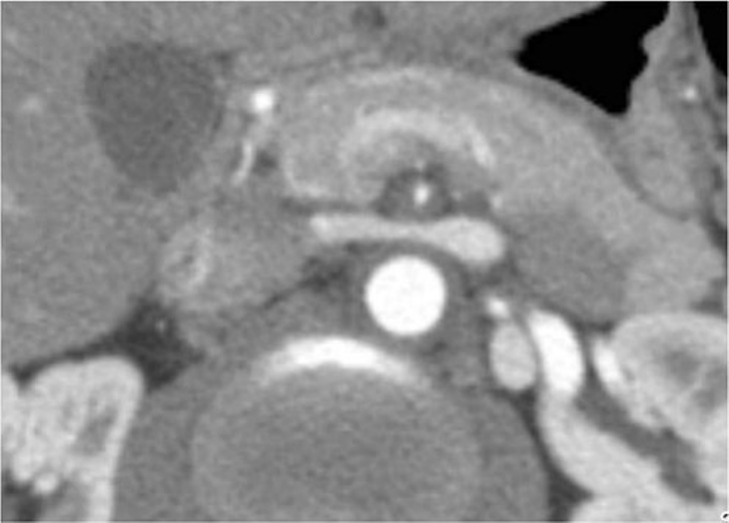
A 36-year-old male with abdominal pain and weight loss over the last 3 months. MDCTA of the abdomen demonstrates diffuse thickening of the aorta and superior mesenteric artery (SMA) with substantial narrowing of the SMA lumen (*arrow*). Courtesy of Dr. Ludmila Guralnik, Rambam Health Care Campus, Haifa, IsraelThat milder degrees of inflammation or wall oedema may not be apparent with CTA is of note. It is considered less sensitive than other modalities such as MR or PET-CT for evaluating the degree of inflammation in the aortic wall [25].Since CTA is performed using iodinated contrast, it offers the additional advantage of allowing rapid exclusion of aortic pathologies that may clinically mimic acute aortitis, including aortic dissection, intramural haematoma and penetrating atherosclerotic ulcer.Chronic stage:Chronic findings include long-standing, burned out aortitis such as linear arterial wall calcification that can be seen after a minimum of 5 years of inflammatory involvement in the aorta or any of the involved vessels, except the ascending aorta [5, 24]. CT can also be used to assess the progression of a potential thoracic aortic aneurysm [1]. High specificity and sensitivity of CT angiography (95 % and 100 %, respectively) [26] as well as high availability make it the imaging modality of choice for the evaluation of TA.Although ionising radiation is of concern, new CT low-dose techniques focussing on decreased kilovoltage and novel reconstruction algorithms provide substantial dose reduction and should be considered, especially when patients are young [27].Magnetic resonance imagingA major strength of MR imaging is its ability to depict wall abnormalities before luminal changes occur [28]. Gadolinium-enhanced fat-suppressed T1-weighted images are preferred to assess thickening and enhancing of the arterial wall and T2-weighted images for showing high signal of the vessel wall representing mural oedema (Figs. 5 and 6). MR angiography may show stenosis at multiple levels, mural thrombi, thickening of aortic valve cusps and pericardial effusions [18, 28–30]. Signal alterations within the pericardial effusion, reflecting fluid and granulation tissue, can be seen as well [18]. Cine MRI can detect cardiovascular and haemodynamic changes, such as aortic regurgitation in patients with TA [31]; MR angiography demonstrates the anatomical location, degree, extent of stenosis and vascular dilation, and patency of collateral vessels and surgical bypass grafts.
